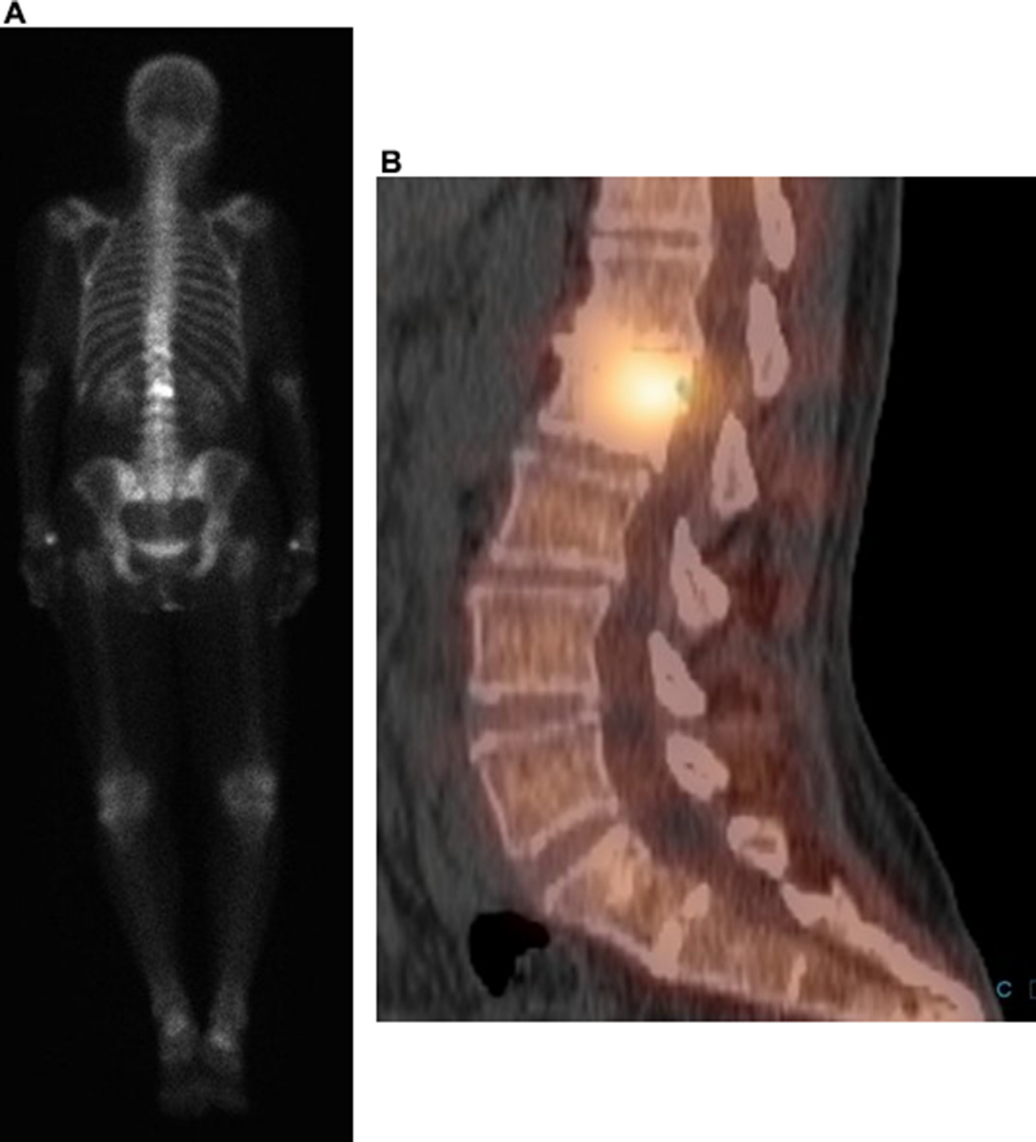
(A) Tc99m MDP whole body bone scan demonstrates focal increased radiotracer uptake in the L2 vertebral body, corresponding to the known lesion. (B) This is confirmed on concurrent SPECT-CT. No additional suspicious osseous uptake is seen on this bone scan.
